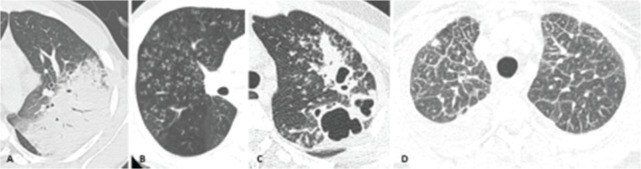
Atypical findings of COVID-19. Axial CT images show (A) isolated segmental consolidation; (B) discrete small centrilobular nodules, some of them with the “tree-in-bud” pattern; (C) lung cavitations; and (D) bilateral smooth interlobular septal thickening with pleural effusion.
